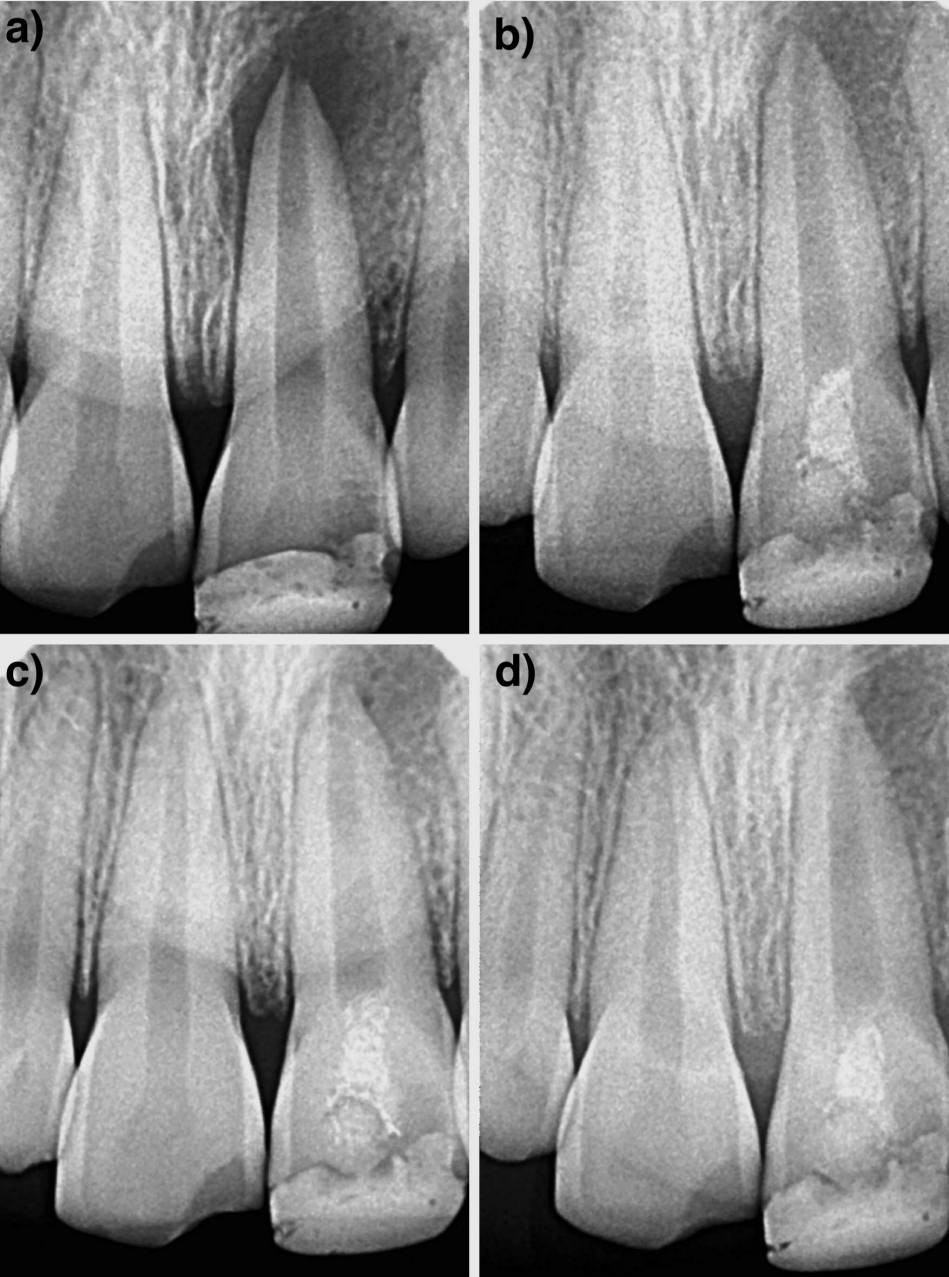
Case 2: Tooth no. 21- (A) Preoperative radiograph; (B–D) Radiographs at 6 months, 12 months and 24 months follow-up period.
